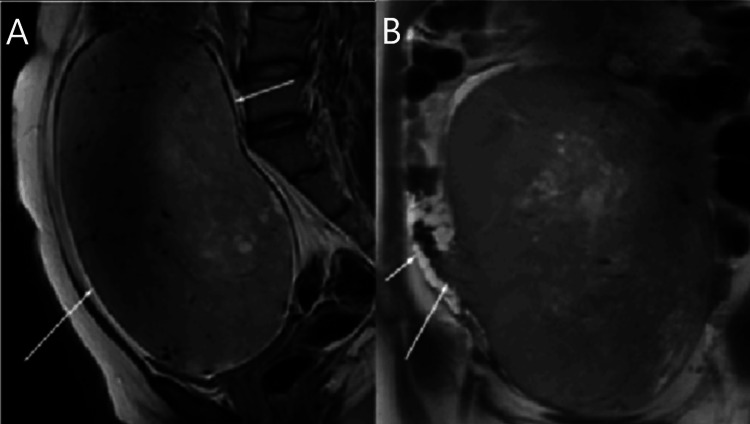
Large abdominopelvic mass of size 12cm x 15cm x 21cm extending up to L4 vertebra and lying close to the anterior abdominal wall MRI abdomen and pelvis (A: Sagittal view, B: Coronal view) revealed a well-circumscribed large abdomino-pelvic lesion of size 12cm x 15cm x 21cm (APxTRANSxCC) extending up to the L4 vertebra and lying close to the anterior abdominal wall.
